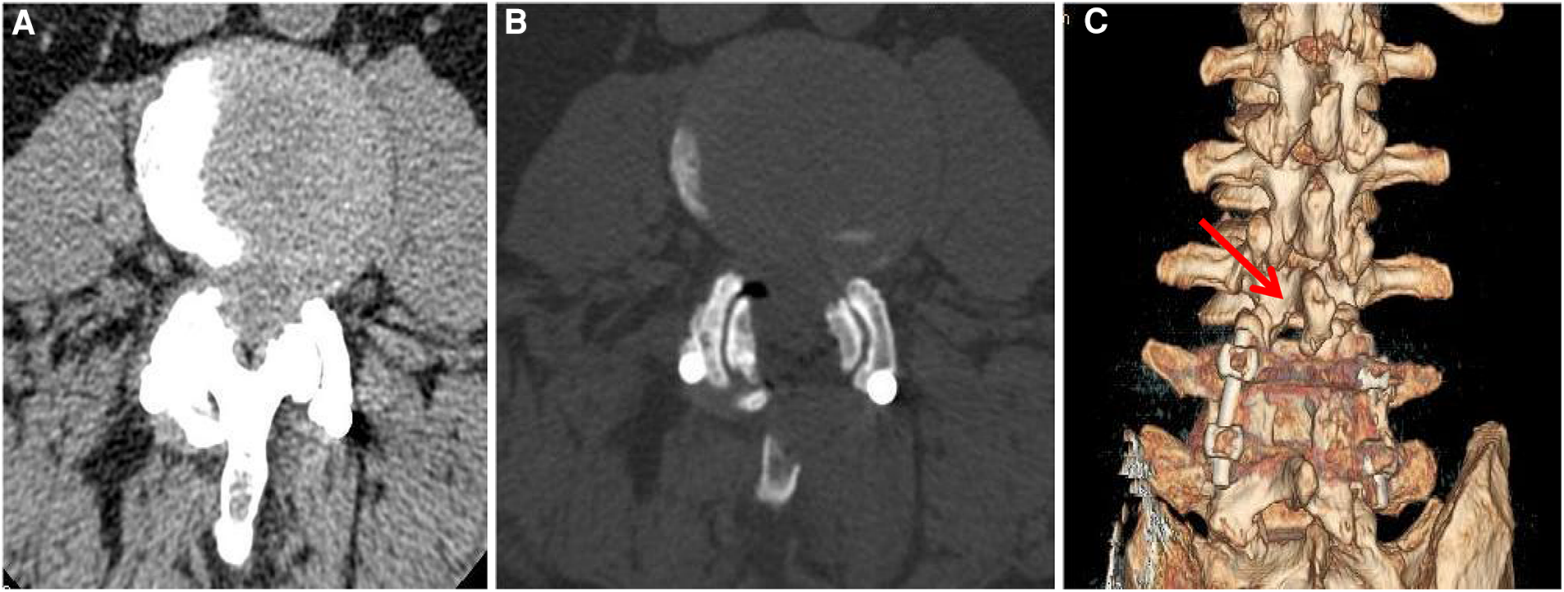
Preoperative and postoperative computed tomography (CT). (A) Preoperative CT showed hypertrophy of the ligamentum flavum at the L3/4 level, hypertrophy of the facet joints, and spinal canal stenosis. (B) Three months after the operation, CT of the lumbar spine showed satisfactory decompression at the L3/4 level, and the facet joints were well preserved. (C) At 3 months after the operation, three-dimensional reconstruction CT of the lumbar spine showed that the facet joints at the L3/4 level were well preserved.
